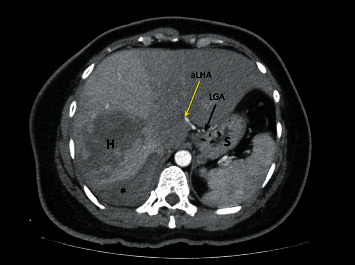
Axial CT images of a patient with a ruptured hemangioma (H) and a subcapsular hematoma (asterix). The left gastric artery (LGA) can be seen medial to the stomach (S). The accessory left hepatic artery (aLHA) originates from the LGA and courses directly into the left liver (type 6 variant).
